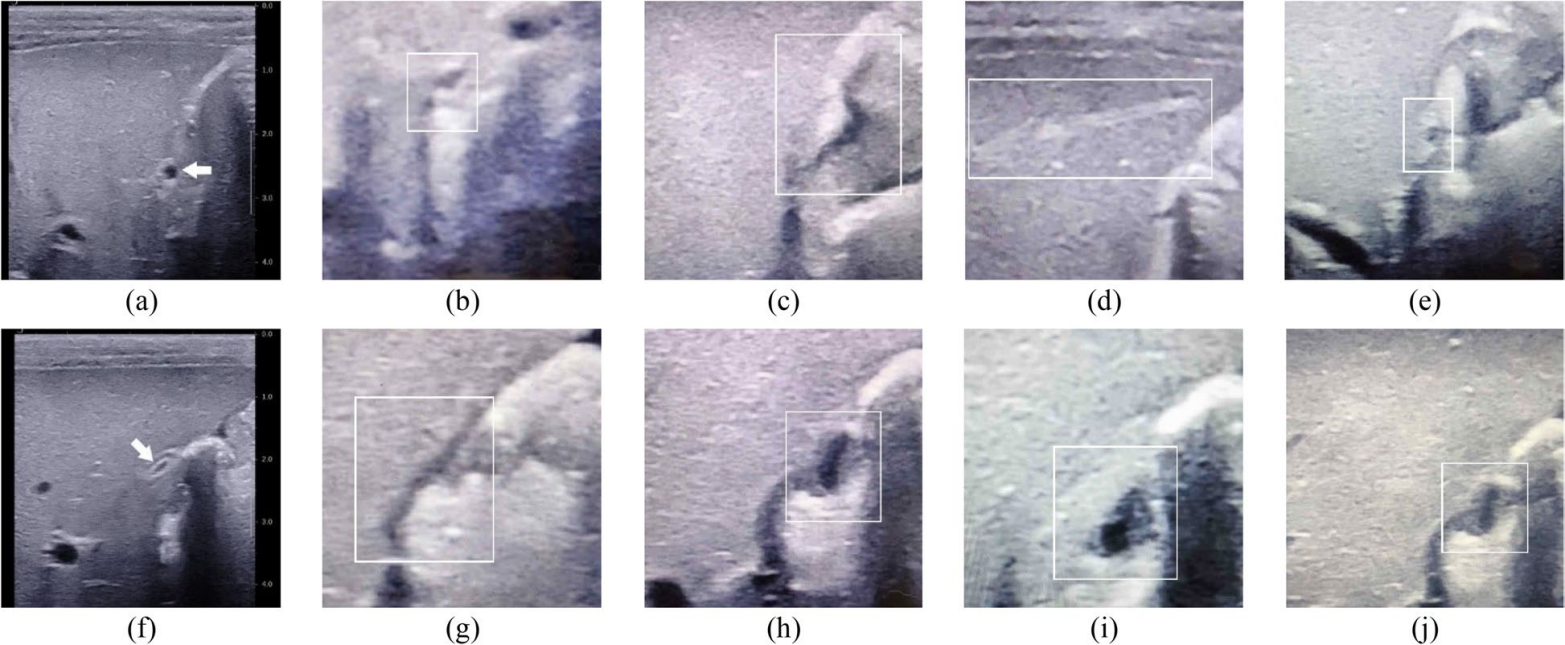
Examples of cases where radiologists consider non-gallbladder as gallbladder. a–e These belong to a 59-day-old female infant with biliary atresia, in which the arrow in a refers to the correct gallbladder (small gallbladder) shown in the original sonographic video. The hepato-intestinal space in b and c, the blood vessels in d, and the gut in e are non-gallbladder structures (rectangles) in smartphone photos, which were considered as gallbladder by four radiologists. f–j These belong to a 58-day-old male infant with non-biliary atresia, in which the arrow in f refers to the correct gallbladder (unfilled gallbladder) in the original sonographic video. The hepato-intestinal space in g and the gut in h–j are the non-gallbladder structures (rectangles) in smartphone photos, which were considered as gallbladder by four radiologists. Sonographic gallbladder videos of these two infants are available in Additional file 4: Video S3 for the first infant and in Additional file 5: Video S4 for the second infant
